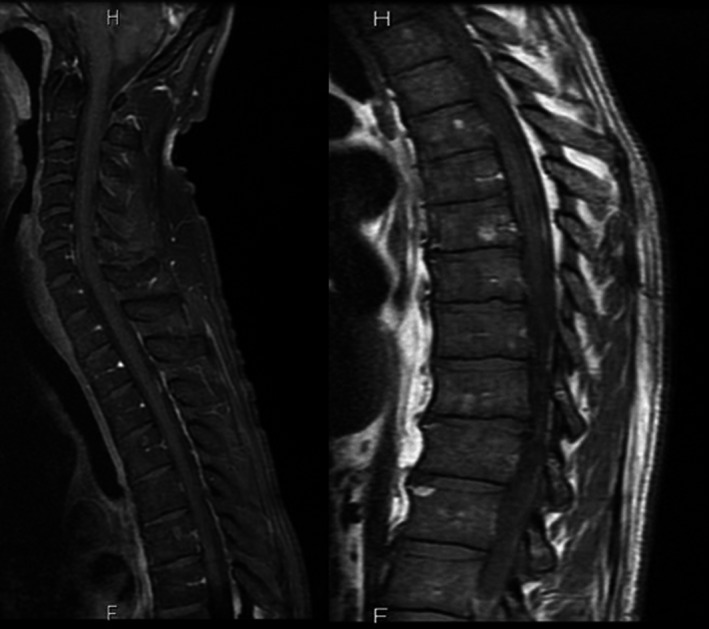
Thoracolumbar spine MRI described diffuse reduced T2‐FLAIR (Fluid‐attenuated inversion recovery) suggesting a deposit disease, as well as vertebral hemangiomas and degenerative changes
